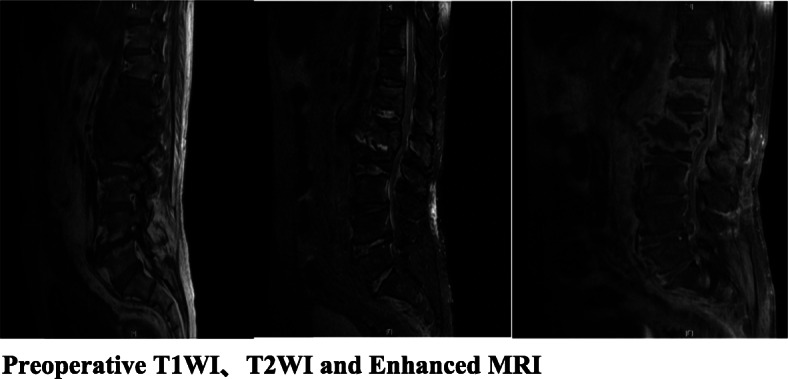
Preoperative T1WI、T2WI and Enhanced MRI
